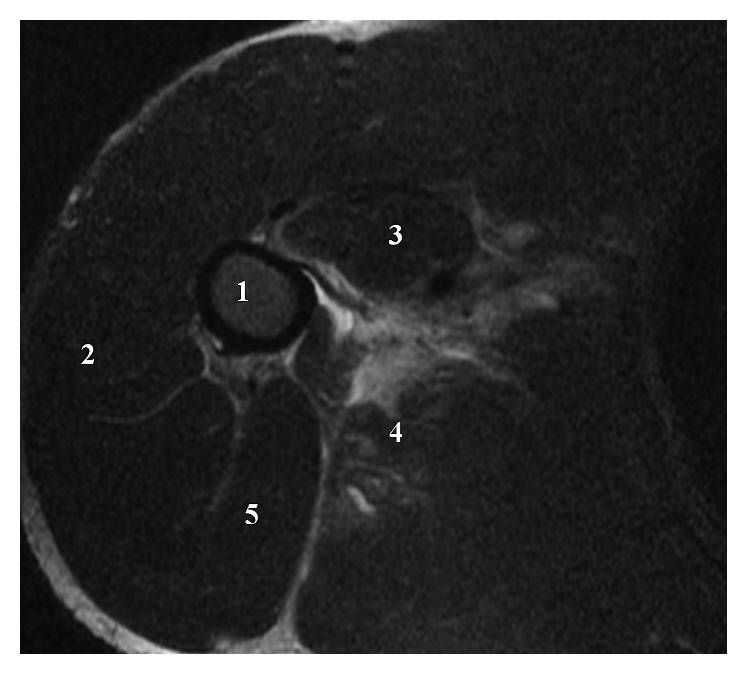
Axial T1 MRI: (1) humerus, (2) deltoid muscle, (3) coracobrachialis muscle, (4) teres major muscle, and (5) triceps muscle.
